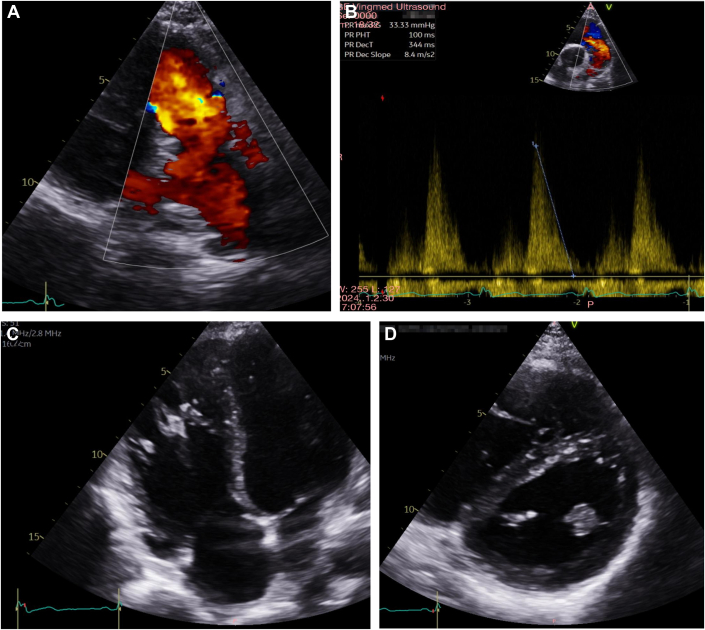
Transthoracic Echocardiogram Prior the Procedure There is free-flow pulmonary regurgitation (a); steep downslope of the continuous wave Doppler of the regurgitation, pressure half time is 100 ms (b); dilated right ventricle on 4-chamber view (c); and diastolic septal flattening on the short parasternal view (d).
